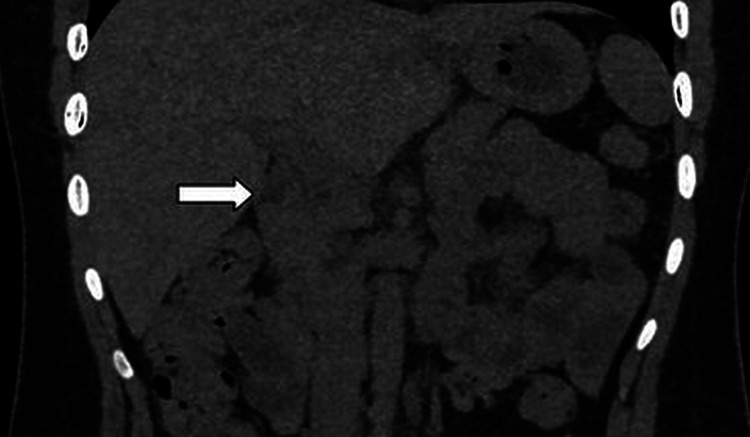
Coronal reformatted non-contrast CT image showing empty gallbladder fossa (arrow). No radio-dense calculi were seen in the biliary system. CT- Computed Tomography
